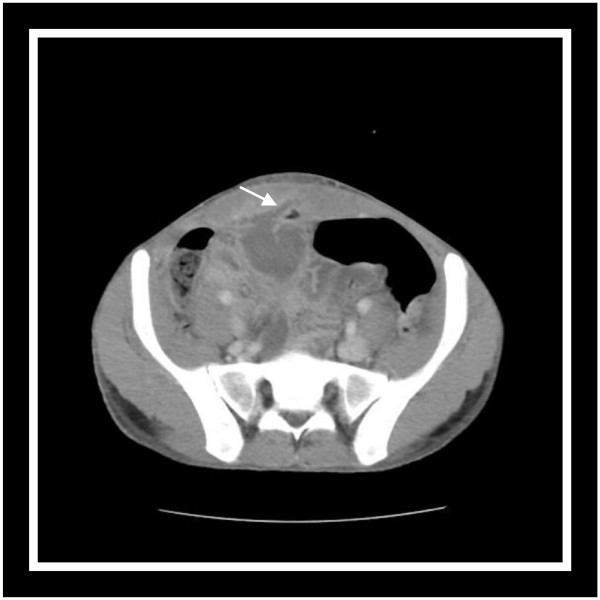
Abdominal computed tomography scan reveals heterogeneous urachal collection communicating with the abdominal wall.
